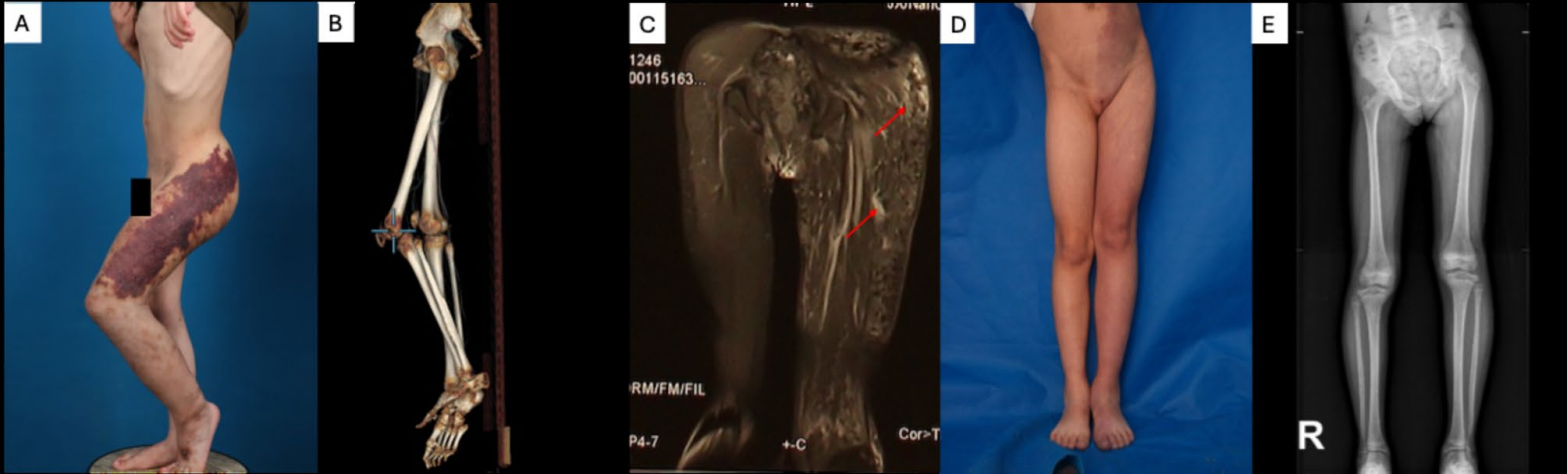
Capillary Malformation with Leg Length Discrepancy(CM-LLD) in Klipple-Trenaunay Syndrome(KTS) and Diffuse Capillary Malformation with Overgrowth(DCMO). A: The Clinical manifestation of KTS: lymphatic blister, capillary malformation, and leg length discrepancy. B: CT scan showed the bone hypertrophy and leg length discrepancy. C: MRI revealed the lymphatic malformation(red arrows show); D: The Clinical manifestation of DCMO: an extensive, diffuse, reticulate capillary malformation with bony hypertrophy; E: Digital radiography of the lower extremities showed longer tibia in the lesional leg than the healthy one
